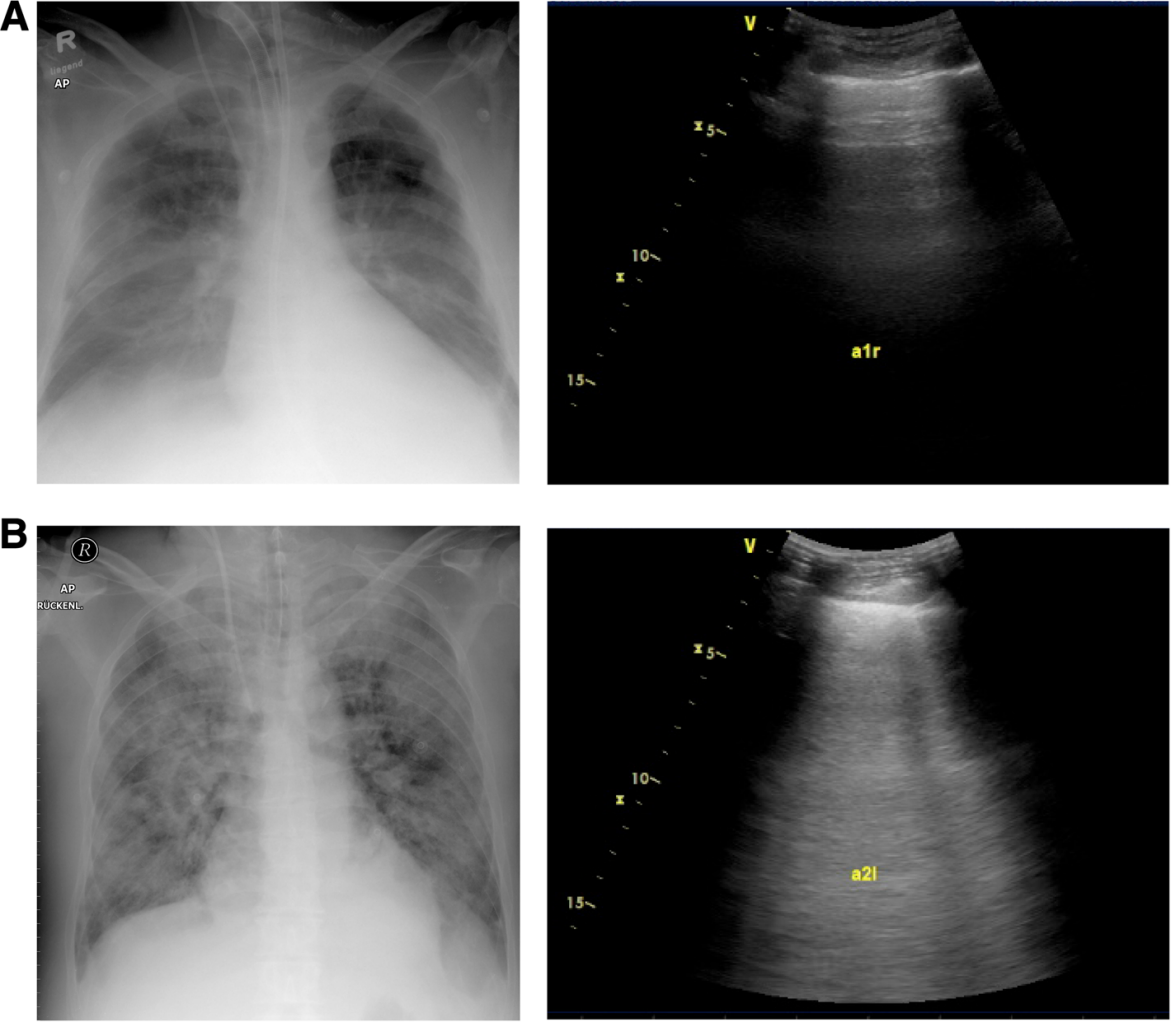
Chest radiographs (left) and corresponding ultrasound screenshots (right) of two study patients. (A) Dry lung with a normal extravascular lung water index (EVLWI) and predominant A lines. (B) Severe, non-cardiac pulmonary edema with a high EVLWI and confluent B lines.
